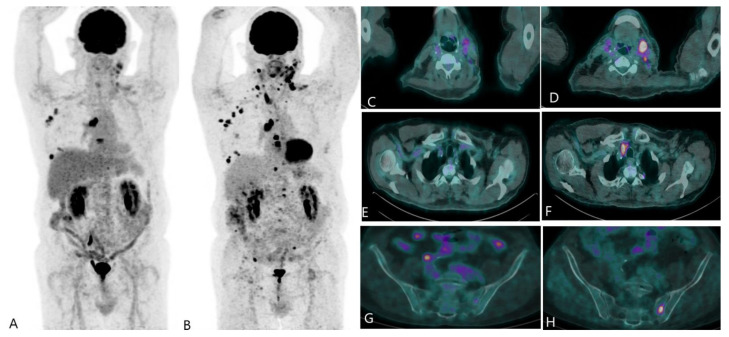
FDG PET/CT in recurrence disease and in evaluation of treatment response. A 65-year-old man was diagnosed with BR five years ago and treated by surgery, chemotherapy, and radiotherapy. He was referred for elevation of tumor marker and left cervical swelling. The PET/CT MIP image (A) shows a recurrence of disease, notably at left cervical, right axillary, and mediastinal lymph nodes, also a bone involvement at T7 and right ribs. The patient was treated then by capecitabine and the evaluation of treatment response was performed 5 months later. The PET/CT MIP image (B) of evaluation showed a progressive disease. Images (C,D) (fusion image, trans-axial view) show the morpho-metabolic progression of cervical lymph nodes. Images (E,F) (fusion image, trans-axial view) show the apparition of a superior paratracheal lymph node involvement. Images (G,H) (fusion image, trans-axial view) show the intensification of uptake of a bone lesion in the left sacrum.
